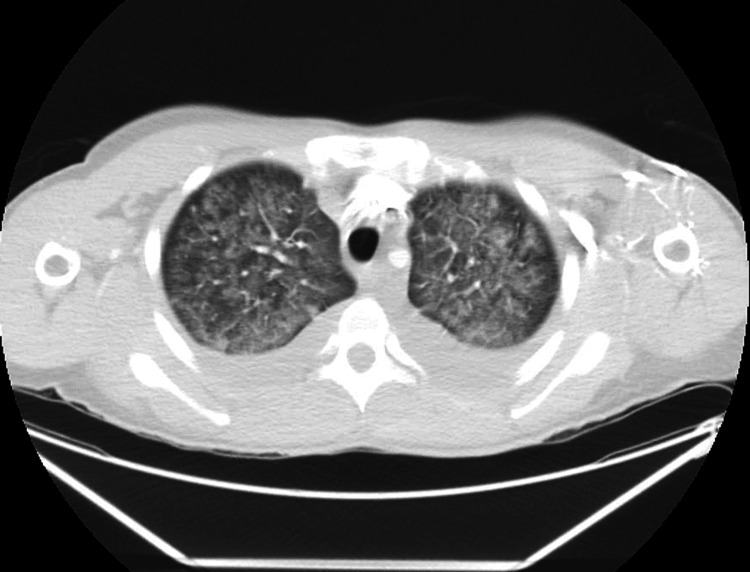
CT of the chest showing diffuse ground-glass opacities in the lungs.
